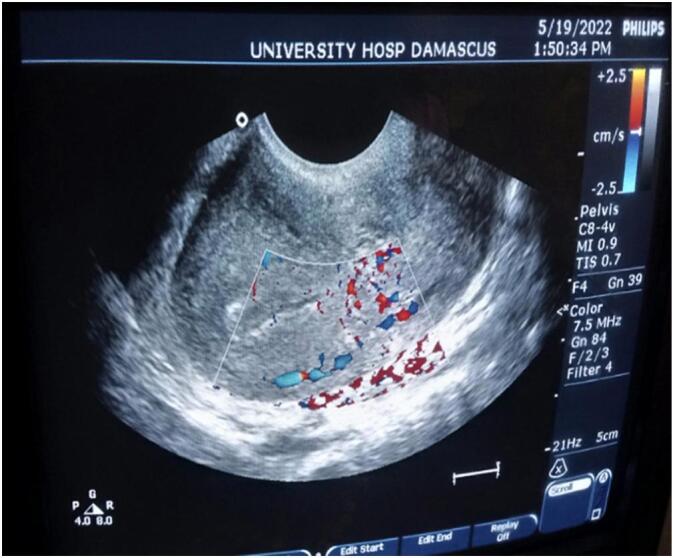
No obvious vascular malformation behind the uterus on TVS with Doppler.
